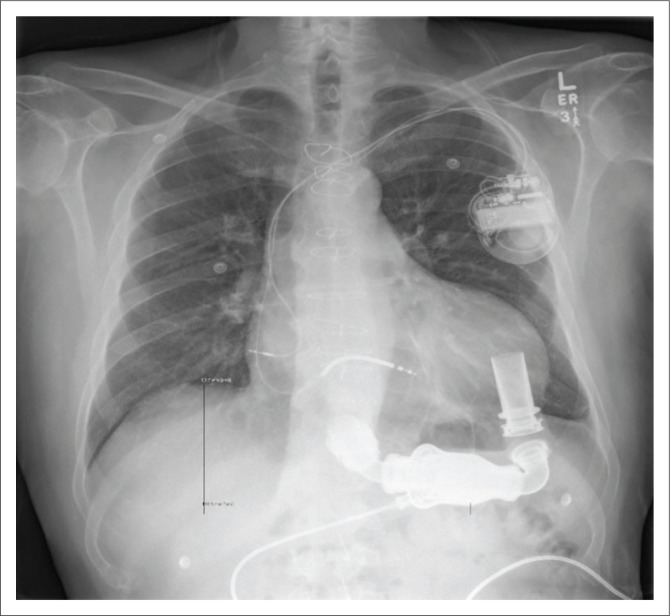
Follow-up chest radiograph after 1 year showed that the same HMII device had migrated cranially by 2 cm.
